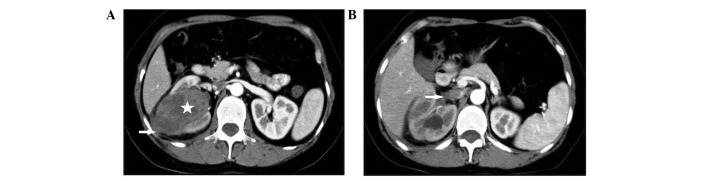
(A) Enhanced computed tomography (CT) image demonstrating a large mass replacing the lower part of the right kidney (star); the mass had invaded the perirenal space and Gerota’s fascia (arrow). (B) Enhanced CT image of the upper abdomen reveals a thrombus in the renal vein and inferior vena cava (arrow).
